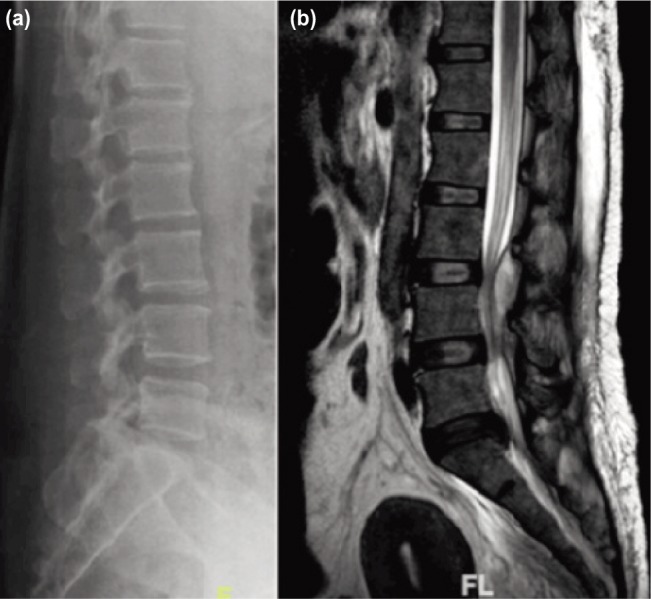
(a) Preoperative plain radiograph lumbo-sacral region, showing no bony involvement. Vertebral bony height and disc spaces were normal, and (b) T2-weighted magnetic resonance imaging sagittal view, showing elongated, diffuse, multisegmented abscess collection causing compression of intraspinal nerve roots at L3/L4 and L4/L5 (cauda equina).
